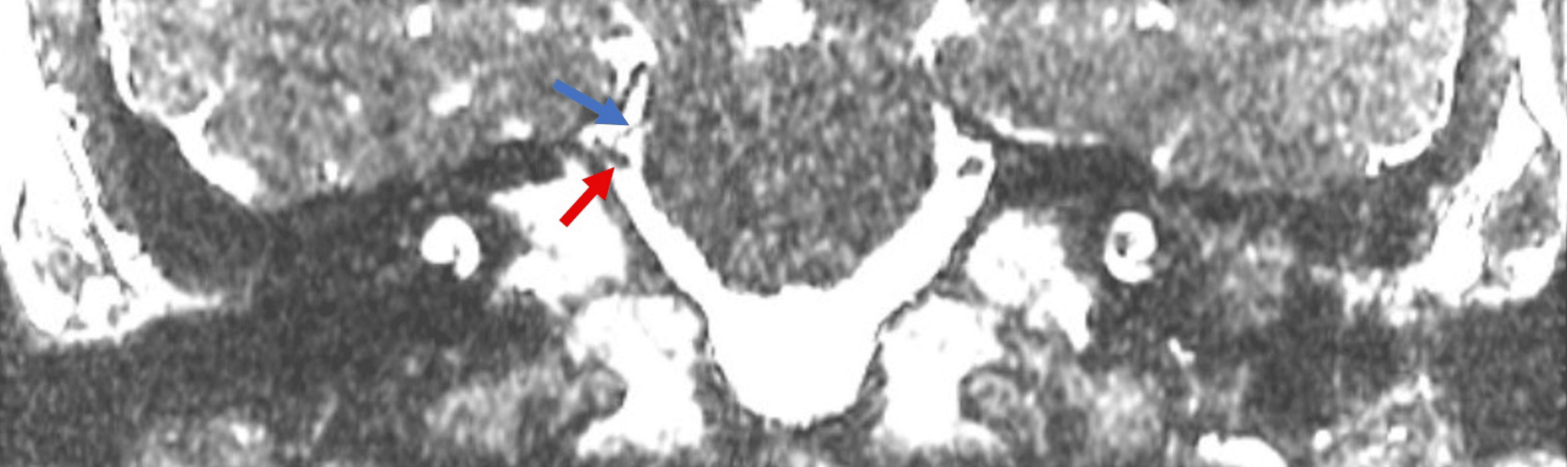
A coronal slice of an MRI brain using the FIESTA protocol. The right superior cerebellar artery (blue arrow) abuts the dorsal aspect of the proximal right trigeminal nerve (red arrow). FIESTA: Fast Imaging Employing Steady-State Acquisition
